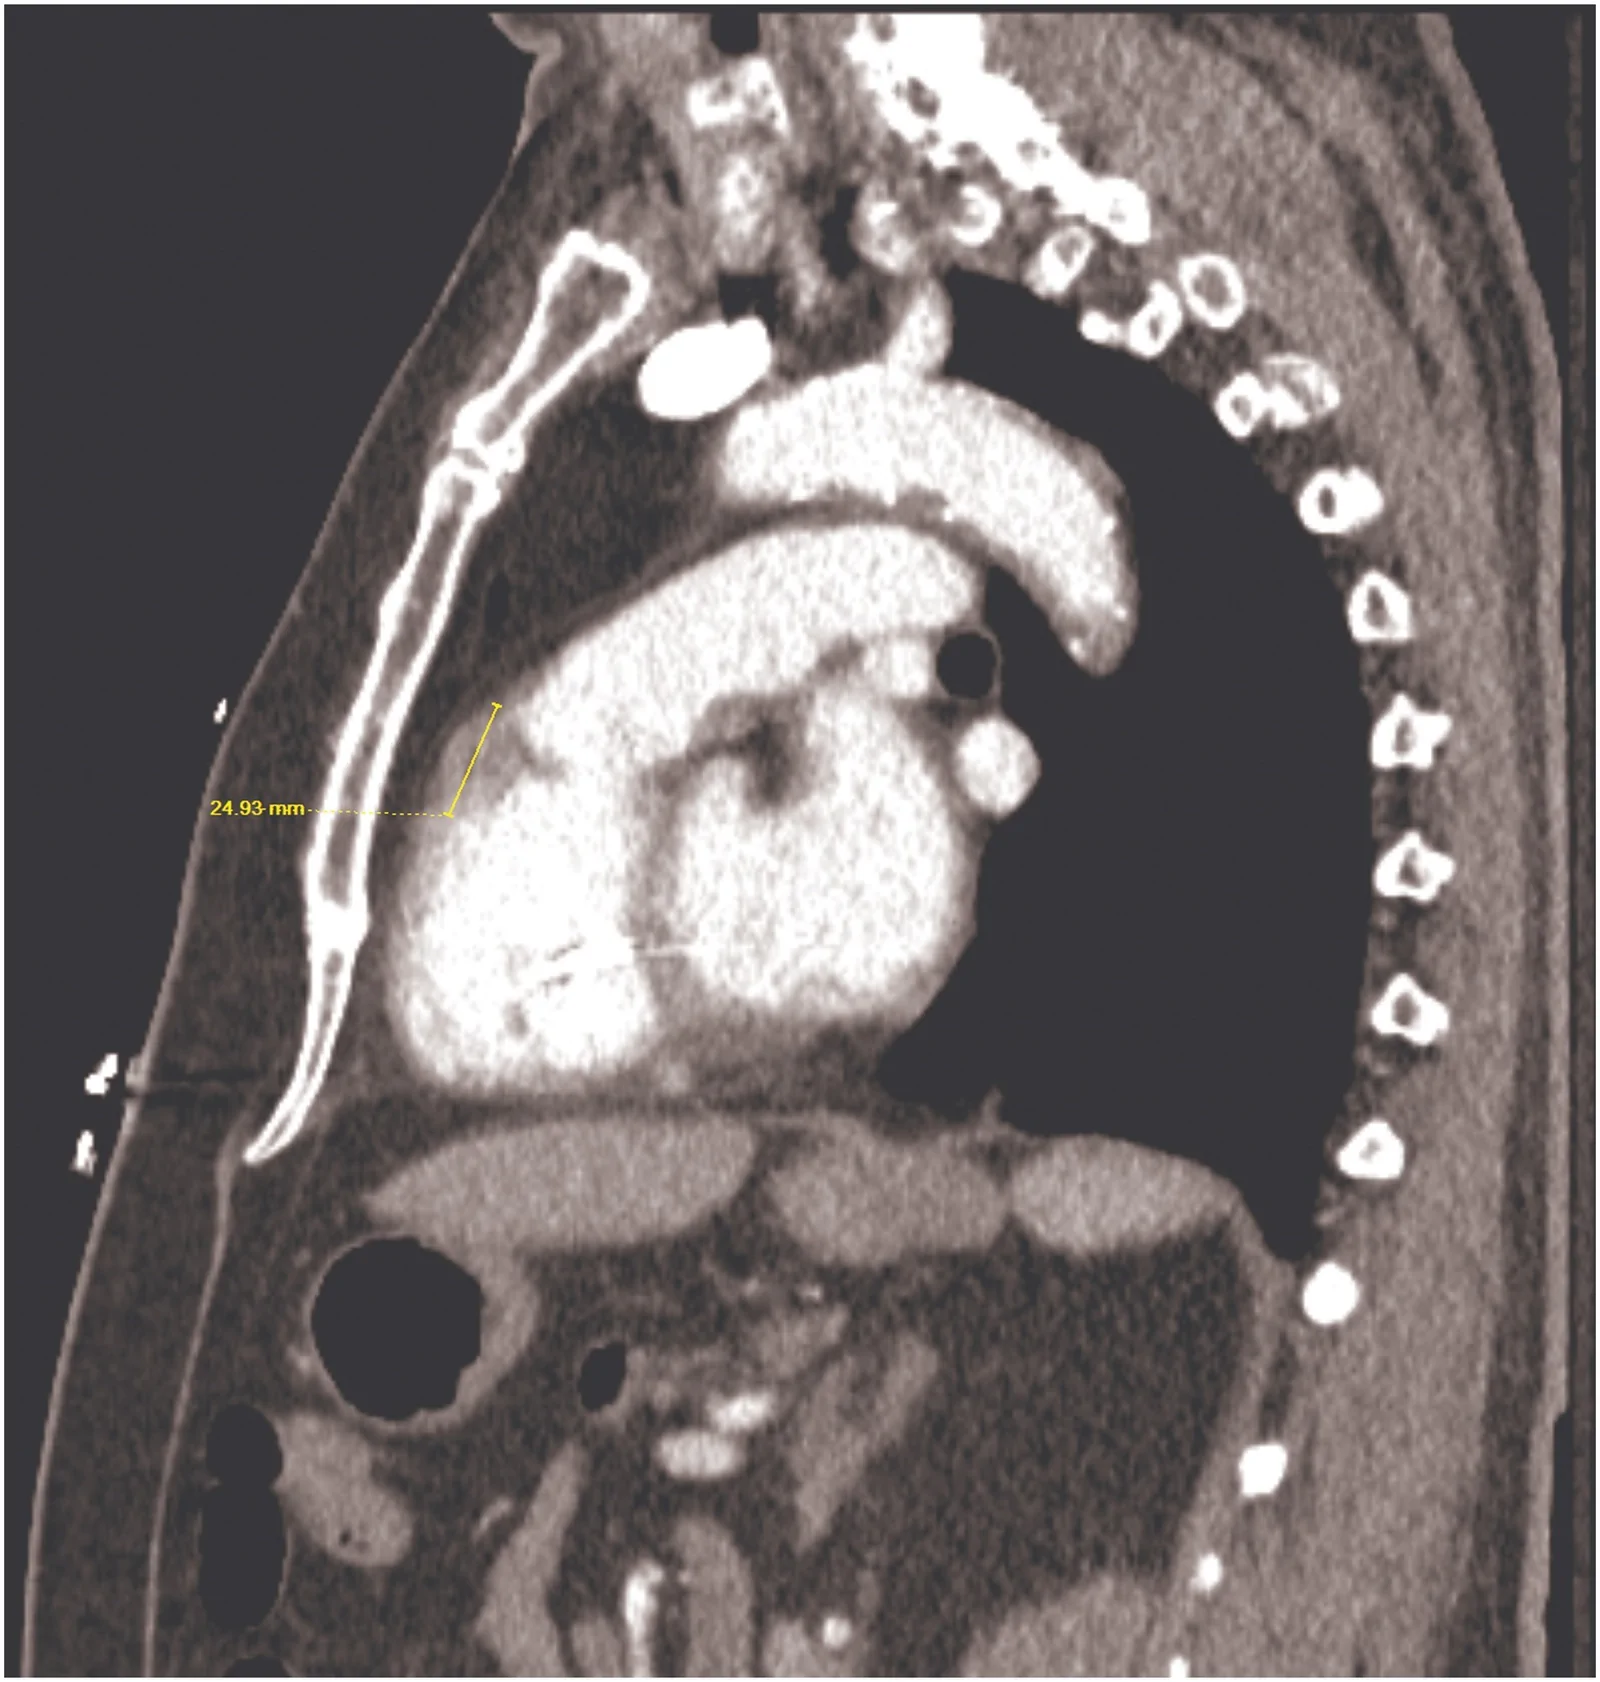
Computed tomography of the chest, sagittal plane. Hypodense filling defect is identified within the right ventricular outflow tract, measuring ∼25 mm in greatest dimension.
